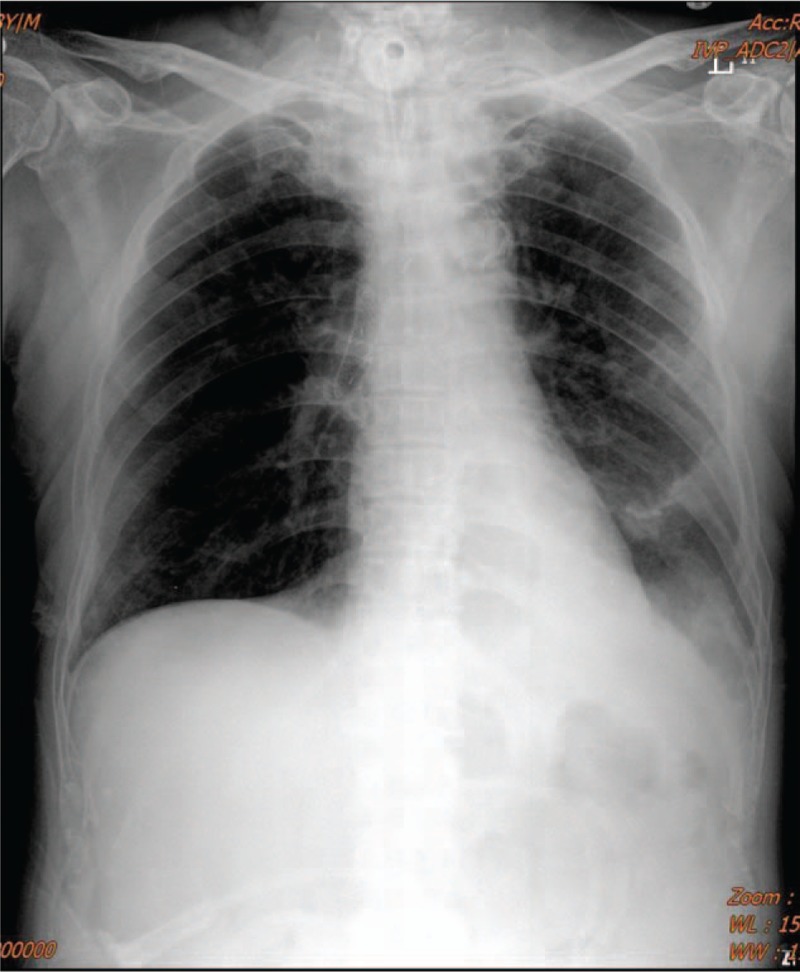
Postoperative chest radiograph shows a stent in right main bronchus with overinflation of right lung and volume reduction of left lung.
